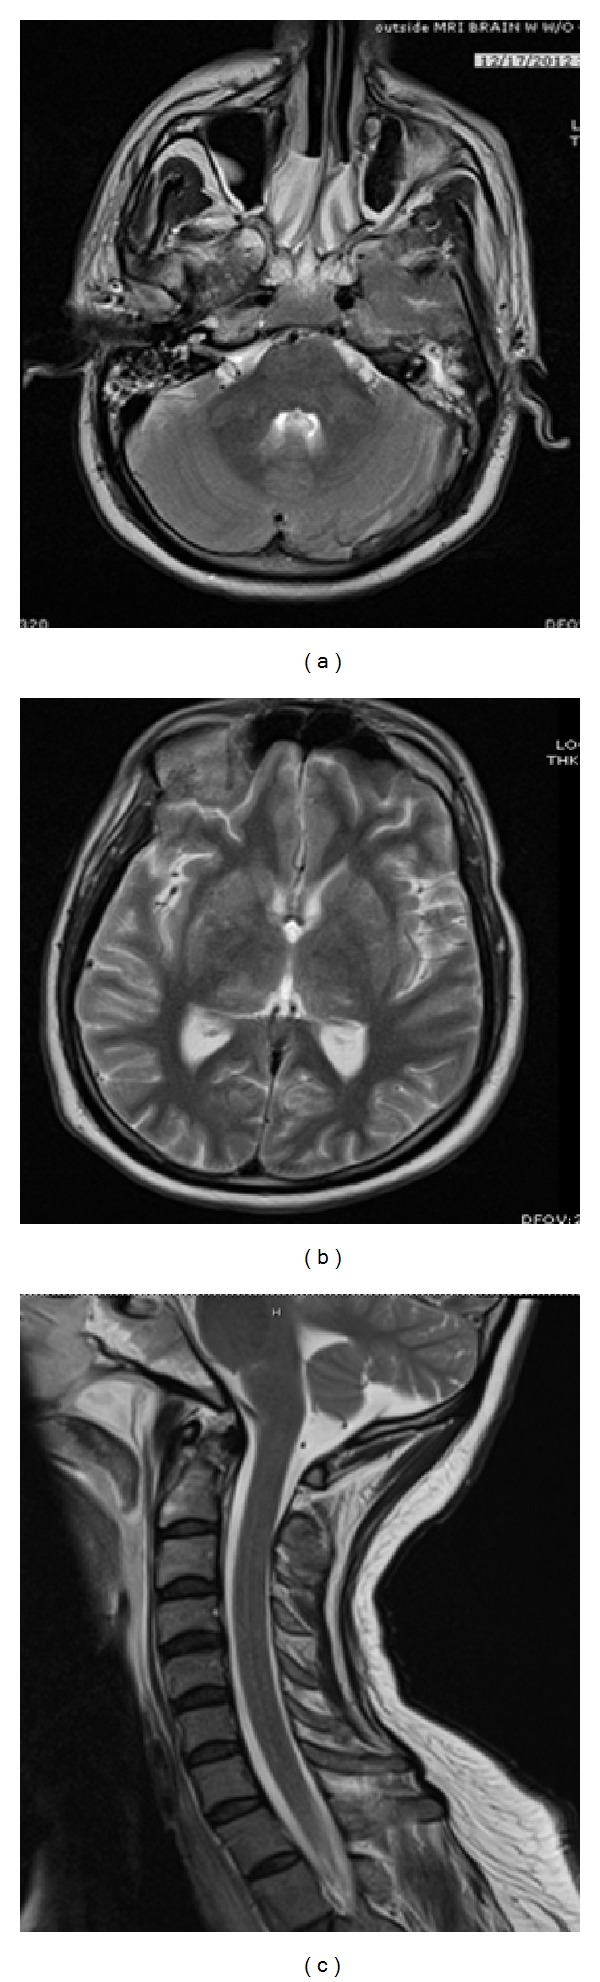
(a) MRI brain showing increased T-2 signal in middle cerebellar peduncles. (b) MRI brain showing increased T-2 signal in bilateral basal ganglia. (c) MRI cervical spine showing mild diffusely increased T-2 signal.
